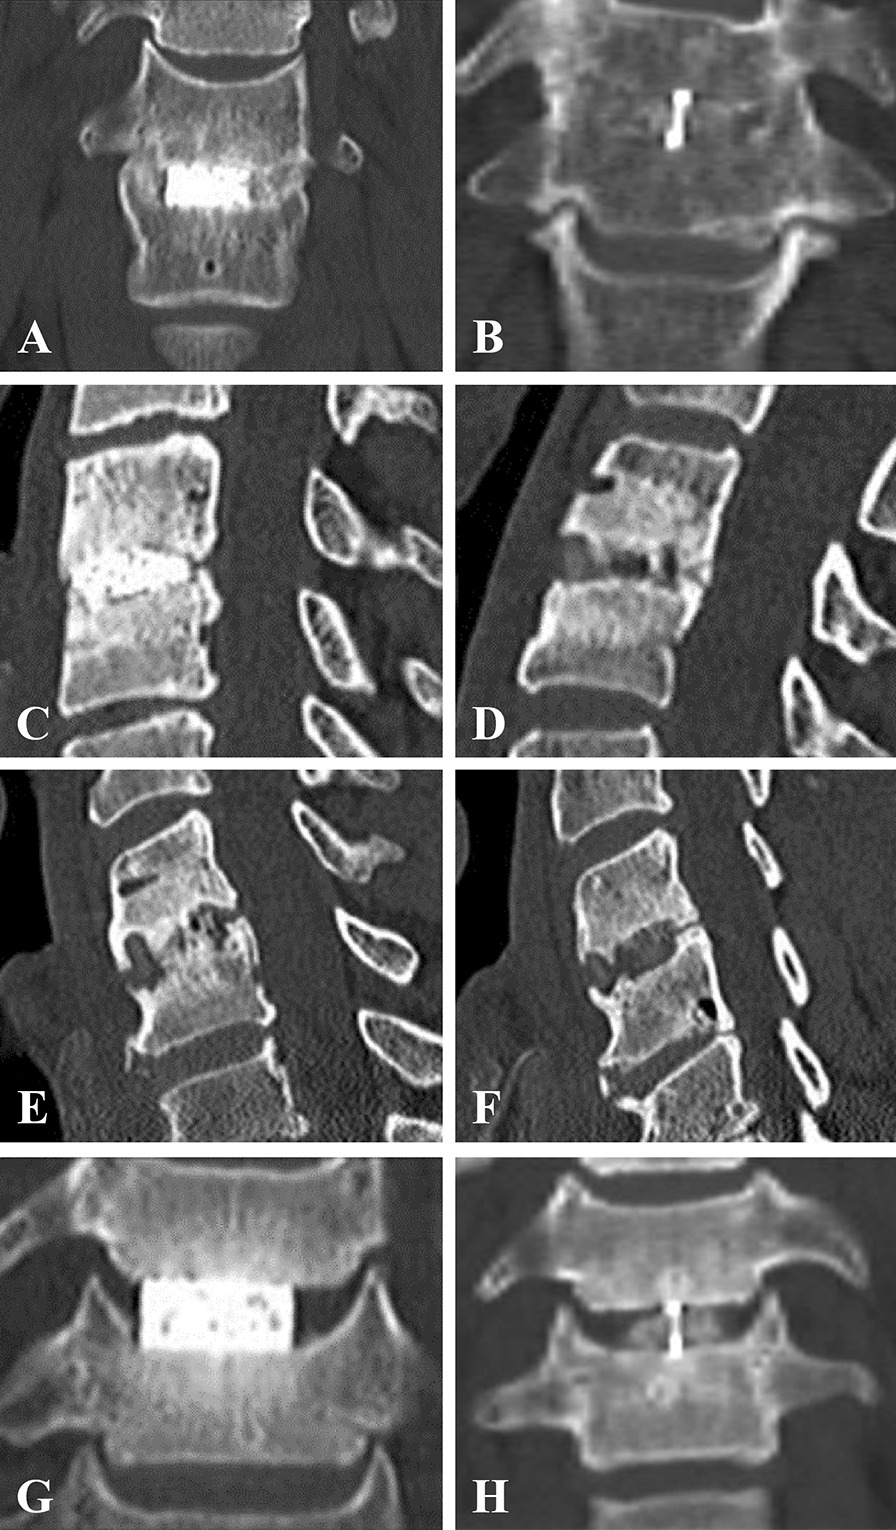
Fusion quality grades on CT scans. Grade I—complete fusion: Al_2_O_3_ cage (A), PEEK cage (B). Grade II—incomplete bipolar fusion: Al_2_O_3_ cage (C), PEEK cage (D). Grade III—unipolar fusion: PEEK cage, with trabecular bridging on the left side (E), no bridging on the right side (F). Grade IV—no signs of fusion: Al_2_O_3_ cage (G), PEEK cage (H)
